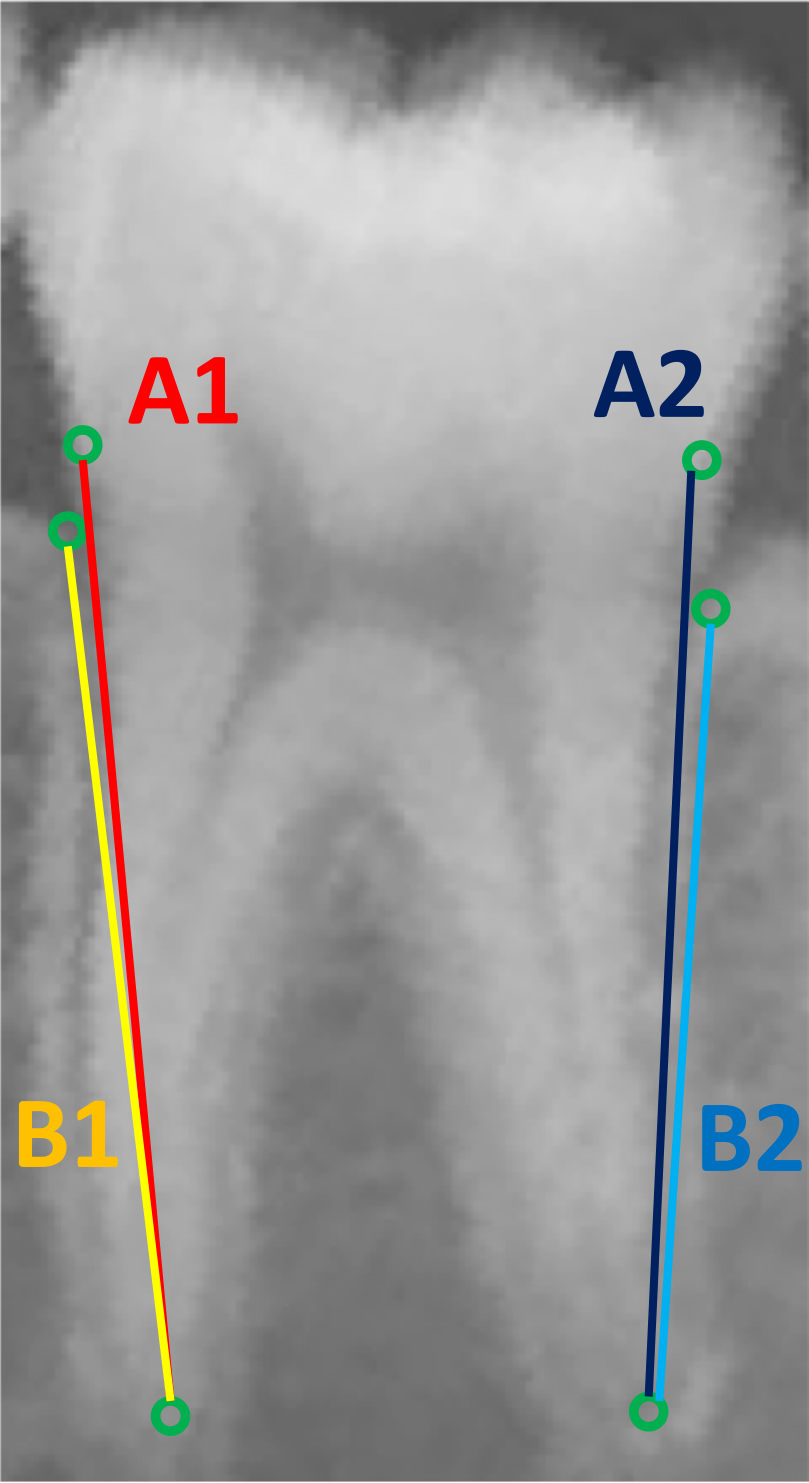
Radiographic PBL calculation. Legend: “Lines drawn between CEJ and root apex points represent root length: A1= left root length, A2= right root length. Lines drawn between the alveolar crest and root apex points represent bone height: B1= left bone height, B2= right bone height. PBL was calculated as follows: PBL 1 (%) = (A1-B1/A1) *100. PBL 2 (%) = (A2-B2/A2) *100”
